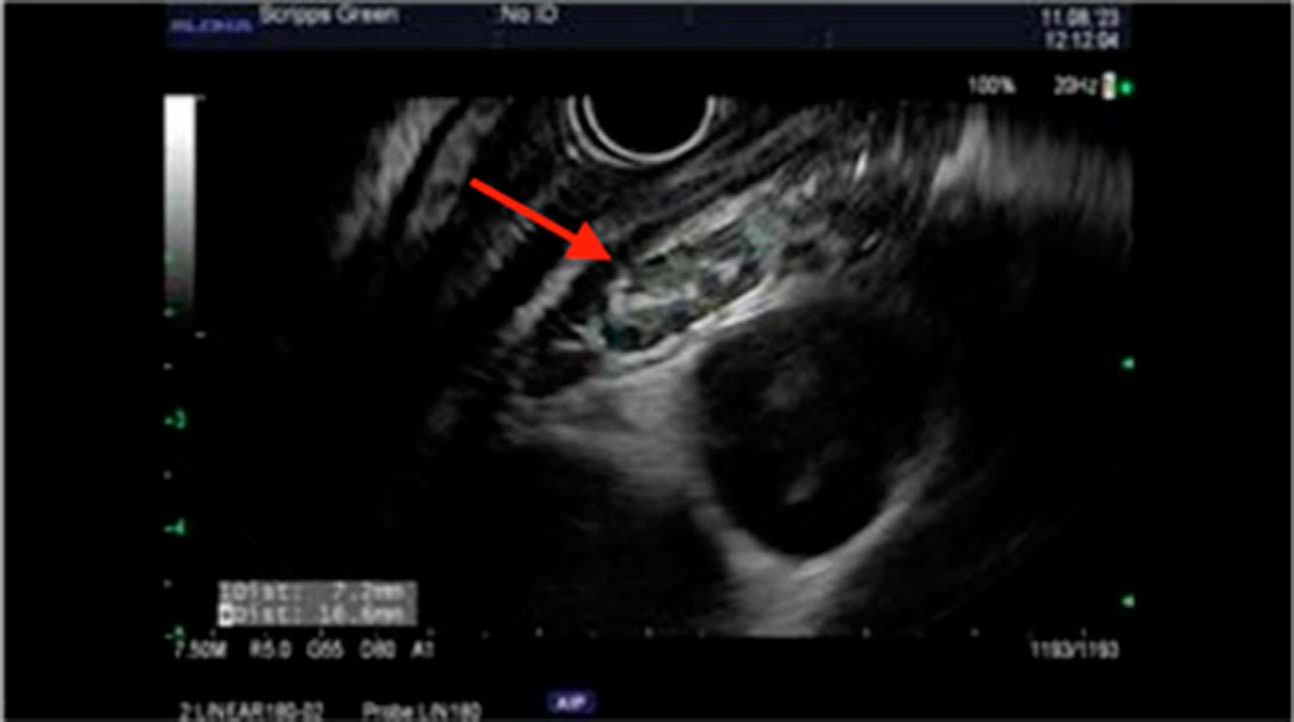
Endoscopic ultrasound notable for circumferential esophageal wall thickening to 8.5 mm. Enlarged lymph node at 25 cm from incisors (red arrow) measured at 18.6 × 6.6 mm status after fine-needle aspiration.
